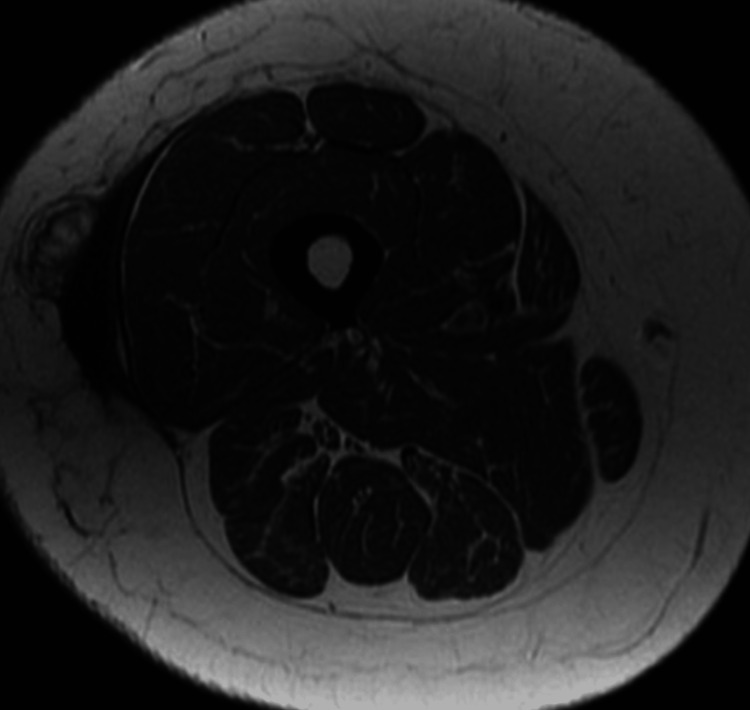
T1-weighted axial MRI through the right mid-thigh showing a hypointense collection in the right lateral thigh.
